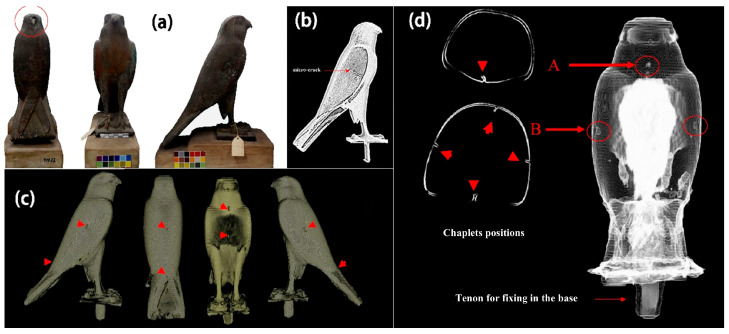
(a) shows god Horus bronze coffin from CEM collection, JE91922; (b) CT topography of the right-wing microfracture; (c) 3D CT images showing indications of the location of small flakes on the outer surface of the Falcon’s coffin reconstructed; (d) showing the location of the core flakes on the inner surface of the Falcon’s coffin, respectively. The letters A and B define two different flap positions [56].
